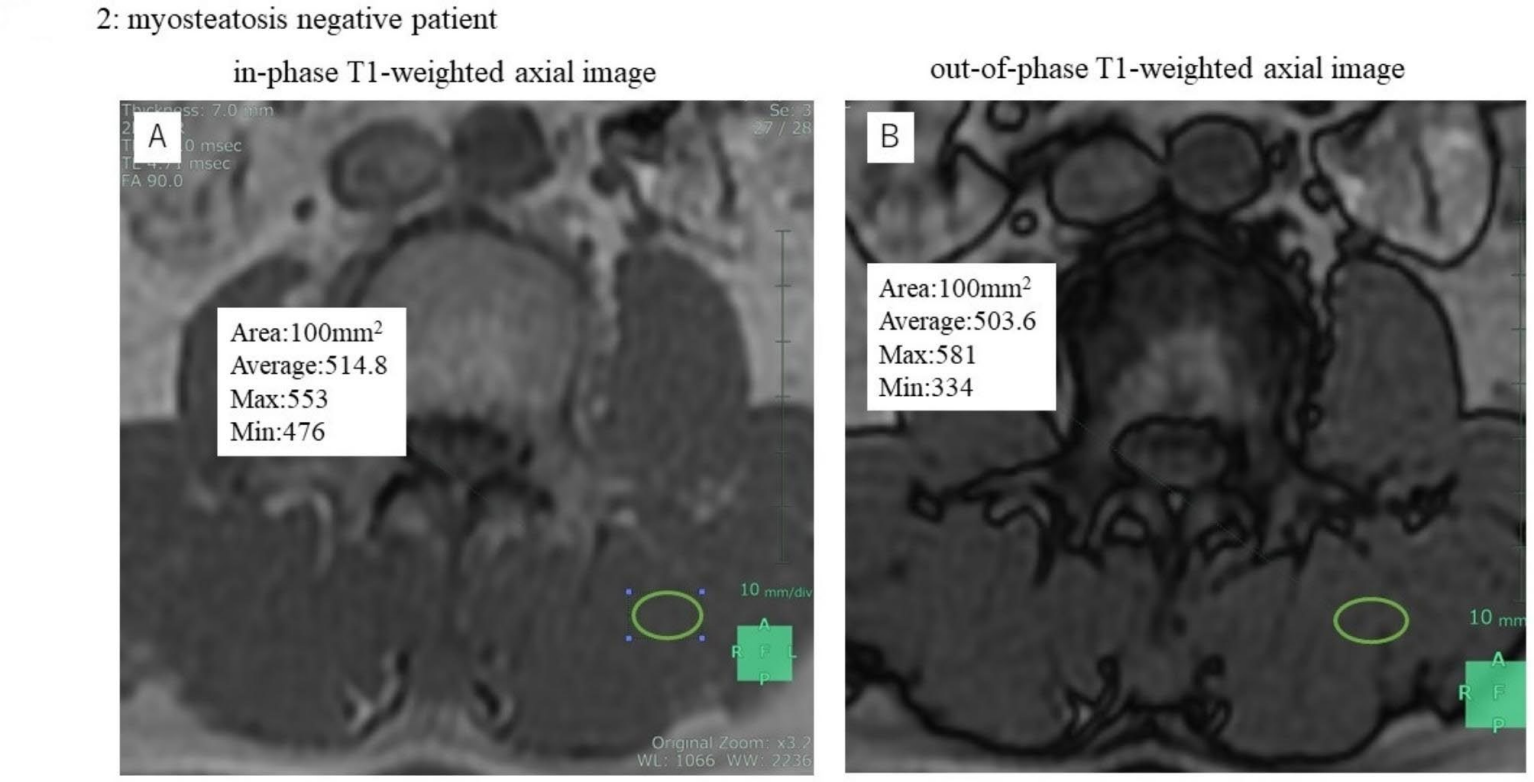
The representative MRI findings in myosteatosis negative patient in-phase T1-weighted axial image out-of-phase T1-weighted axial image
